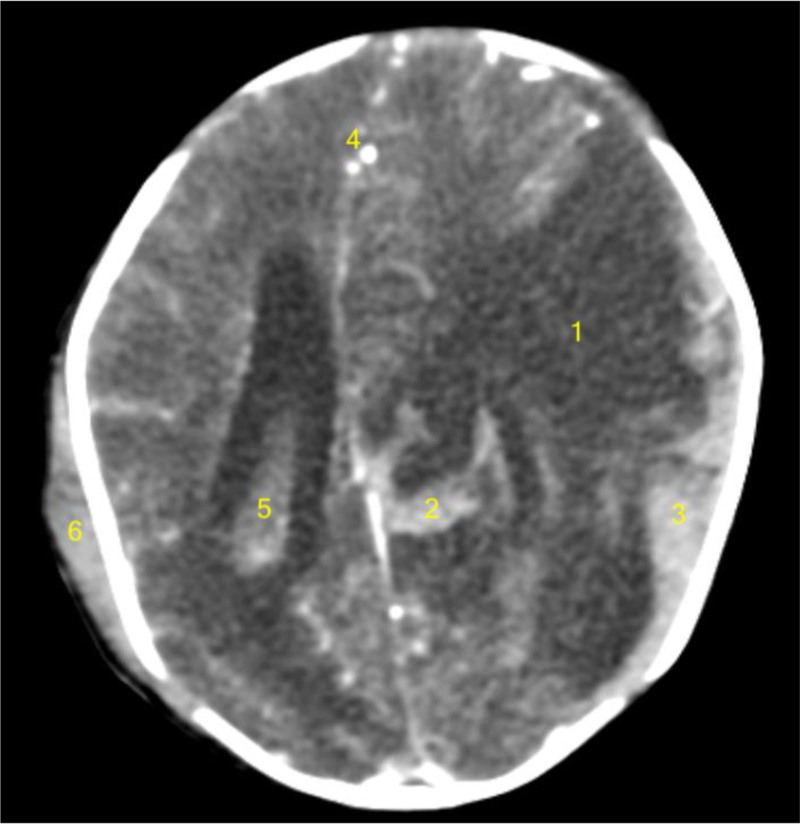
Brain CT at 2-day-old, axial section, brain window. A large area of acute brain tissue edema mixed with hemorrhage in the left frontal-temporal-parietal lobes and basal ganglion, acute ischemic stroke (1) with hemorrhagic transformation (2) of the left MCA territory. There was also a large acute subdural hematoma (3) in the left frontal-temporal-parietal region. This causes a strong mass effect, compressing the left lateral ventricle and shifting the midline to the right side (4). There was also intraventricular hemorrhage (IVH) in all the ventricles (5) with associated hydrocephalus, and severe subcutaneous swelling with hemorrhage (6) in the right frontal-parietal region. CT = computed tomography, IVH = intraventricular hemorrhage.
